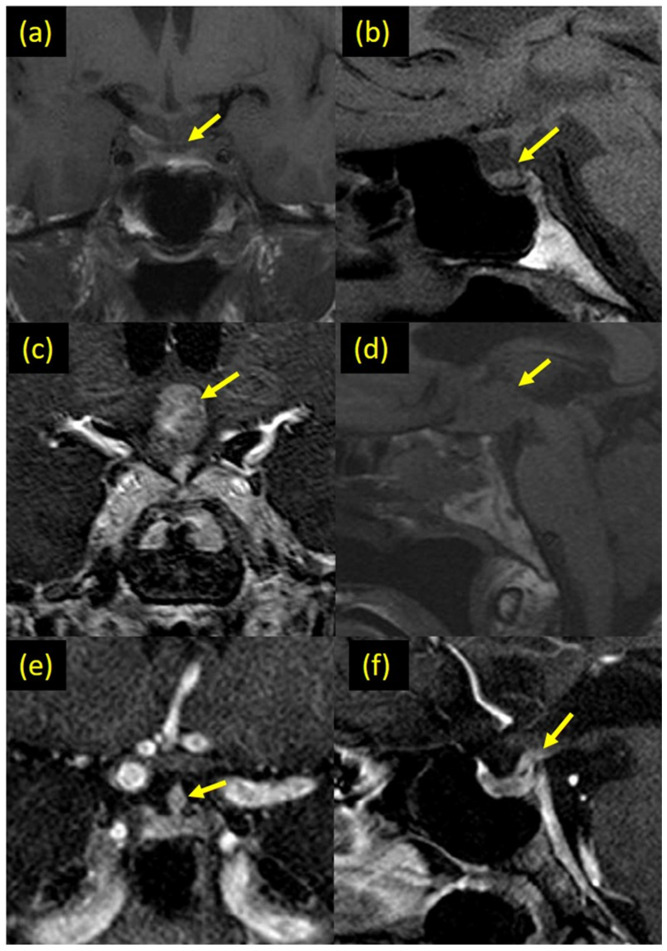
(a,b) T1 weighted MRI images of the skull base showing a small size pituitary gland with significant narrowing of the pituitary stalk. (c) Coronal T1-weighted post-contrast and (d) sagittal T1-weighted pre-contrast MR-images showing suprasellar post-chiasmatic mass involving the pituitary stalk and the hypothalamic floor, which is intensely enhanced in the post-contrast images. (e) Coronal T1-weighted post-contrast MR-image showing focal engorgement of the middle segment of the pituitary stalk due to ECD infiltration (early ECD involvement of the pituitary stalk. (f) Sagittal T1-weighted post-contrast MR-image showing abnormal thickening of the most proximal end of the pituitary stalk, at the point of its insertion into the hypothalamic floor.
